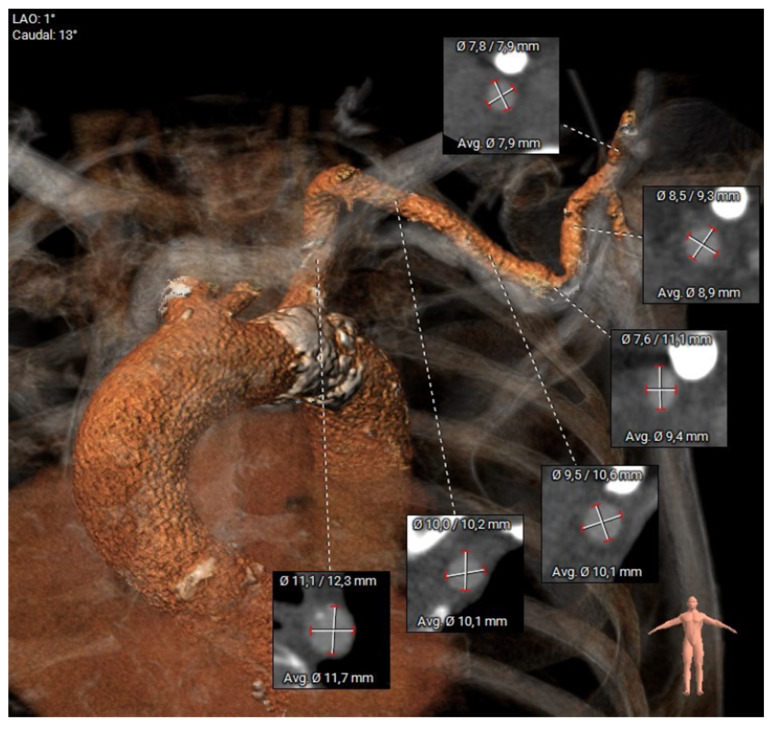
CTA reconstruction of aorta and axillary artery with vessel diameters. Calcification load can be identified.
